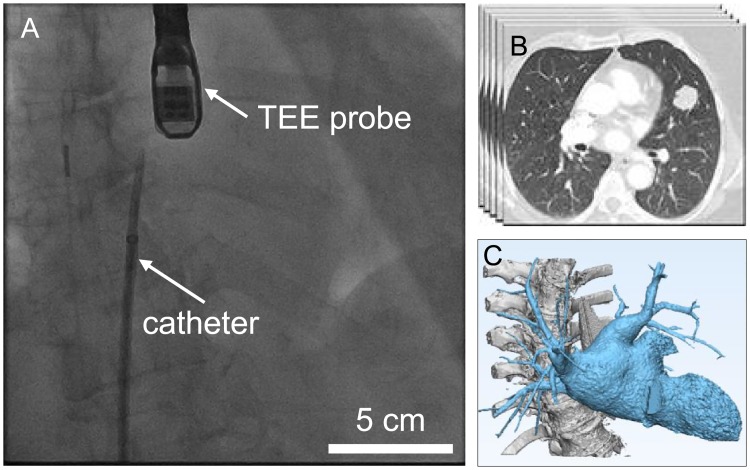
Imaging modalities in cardiovascular interventions. A: The heart is transparent in real-time X-ray fluoroscopic images. B: Pre-operative CT scan images provide a high contrast for understanding heart anatomy with (C) the 3D reconstruction.
